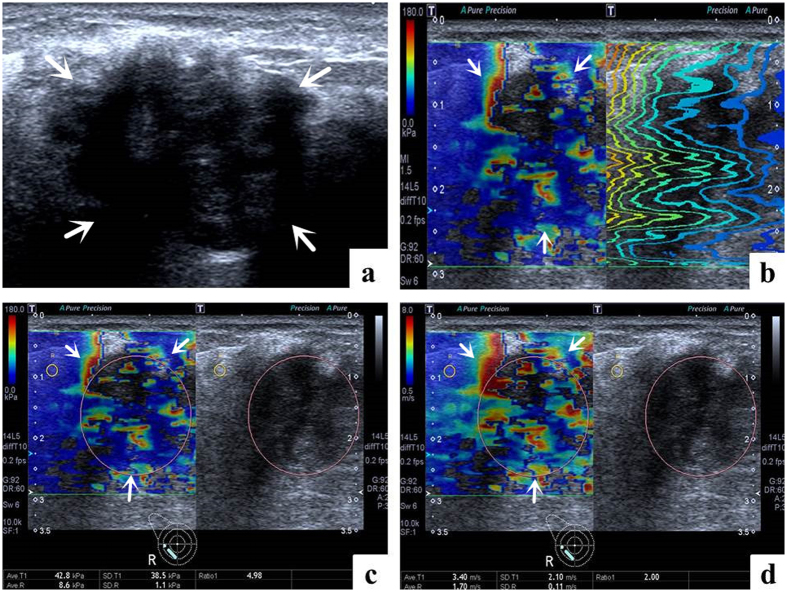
Images in a 62-year-old woman with invasive ductal carcinoma. (a) The lesion (arrows) is shown on B-mode ultrasound. (b) The lesion (arrows) shows irregularly distorted and chaotic contour lines on the shear wave propagation mode. (c) The Emean, E_SD_ and Eratio of the lesion (arrows) on elasticity mode are 42.8 kPa, 38.5 kPa and 4.98 respectively. (d) The Emean, E_SD_ and Eratio of the lesion (arrows) on shear wave speed mode are 3.40 m/s, 2.10 m/s and 2.00 respectively.
